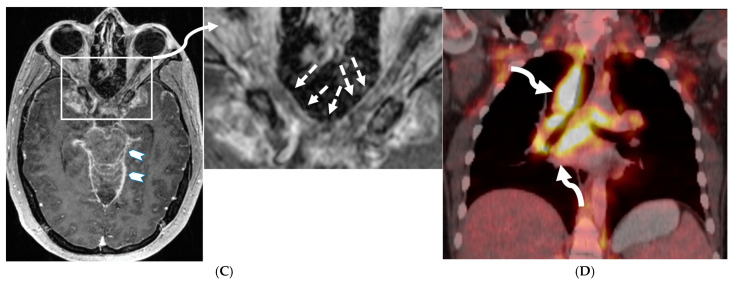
18-year-old presented with headache, persistent vomiting and weight loss. History of sarcoidosis diagnosed 2 years ago. Axial Fluid Attenuated Inversion Recovery (FLAIR) (A), Axial T1 post contrast (B), Axial T1 inversion recovery post contrast (C) and Coronal Positron Emission Tomograpy (PET) scan (D): There is a heterogeneously enhancing ill-defined area of T2/FLAIR hyperintensity involving the medial aspect of the left globus pallidus (arrows), anterior aspect of the left thalamus and left hypothalamic region. Diffuse enhancement of the basal meninges, tentorium, throughout perisylvian sulci (arrow heads), along the infundibulum, and posteriorly at the craniocervical junction. There is also enhancement along optic nerve sheath (dashed arrows). Features are highly consistent with extensive neurosarcoidosis given the previous history of thoracic sarcoid. PET scan from 2 years earlier demonstrating avid uptake of radiotracer (curved arrows). Radiologically, the differential diagnosis includes tuberculosis and metastatic process. Patient made complete recovery after treatment for sarcoid.
